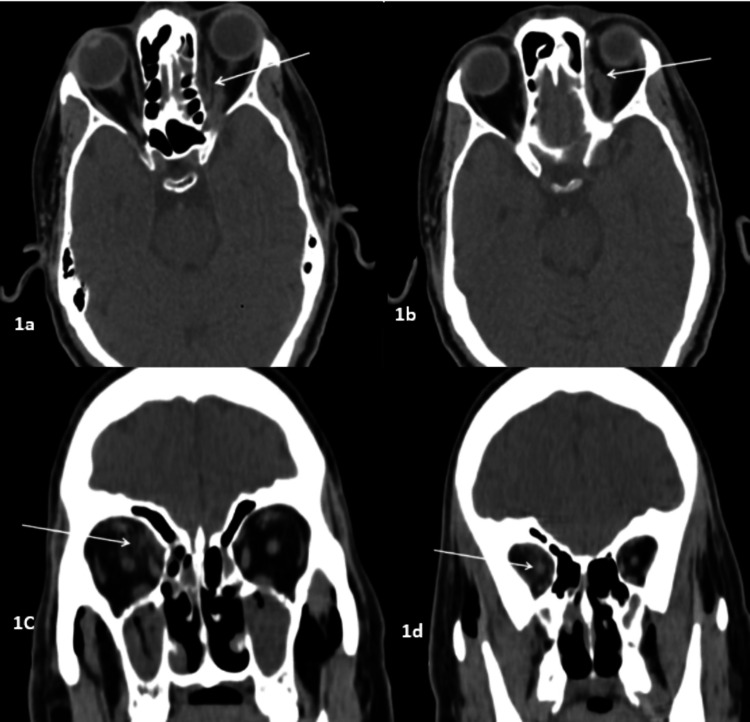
Orbital involvement in different patients 1a and 1b: Axial CT image of a 57-year-old diabetic patient with ROCM showing left intra- and extraconal fat stranding with soft tissue opacification of the left orbital apex (arrow) and thickening of the left superior ophthalmic vein (arrow). 1c and 1d: Coronal CT scan of another 68-year-old patient with ROCM showing right intra- and extraconal fat stranding (arrow) with thickening of the right optic nerve (arrow). CT: computed tomography, ROCM: rhino-oculo-cerebral mucormycosis
